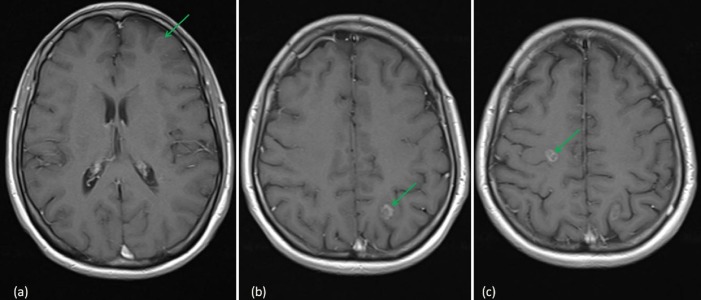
MRI head following WBRT (March 2015) (a) 7.3 x 6.8 x 5.2 mm left frontal BM; (b) 9.6 x 9 x 10.7 mm left parietal BM; (c) 9.4 x 7 x 11.5 mm right frontal BM MRI: magnetic resonance imaging; BM: brain metastases; WBRT: whole brain radiotherapy
